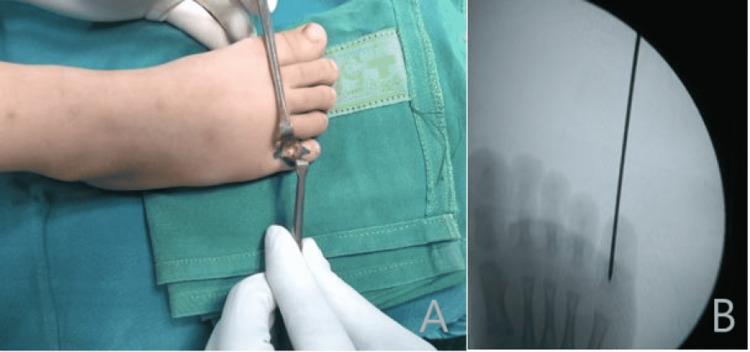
Intraoperative photograph and radiograph Exposure of the dorsal aspect of the proximal interphalangeal joint (panel A) and anatomic reduction of the fifth digit proximal interphalangeal joint with Kirschner wire stabilization (panel B)
